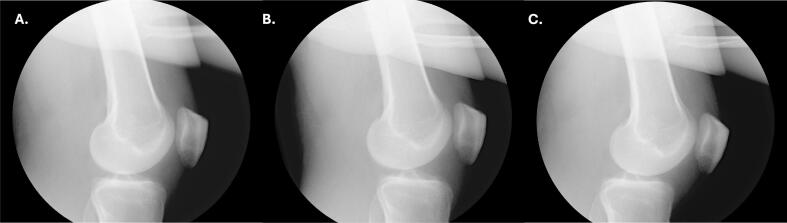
Lateral radiographs of the right knee during EUA without applied stress (A), with anteroposterior stress applied demonstrating anterior translation of the tibia (B), and with anteroposterior stress applied demonstrating posterior translation of the tibia (C). views of the right knee at the final follow-up visit, ten months after the injury.
